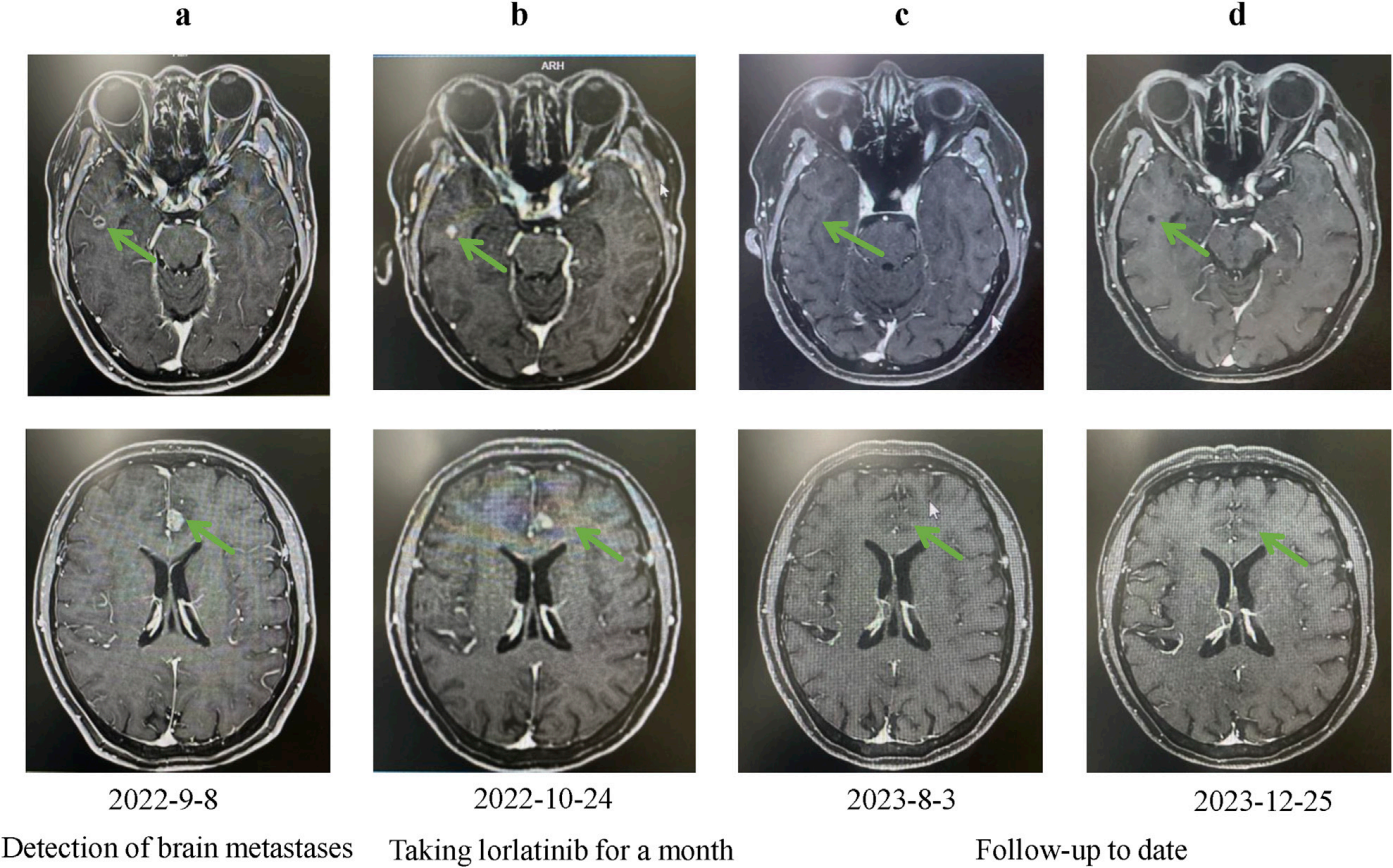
Changes in brain lesions during Lorlatinib treatment. (A) Baseline MRI showing brain lesions on 2022-9-8; (B) Follow-up MRI after one month of Lorlatinib treatment on 2022-10-24, showing partial reduction in lesion size; (C) Further improvement observed on MRI after sustained treatment on 2023-8-3; (D) Continued regression of brain lesions on MRI after long-term treatment on 2023-12-25.
